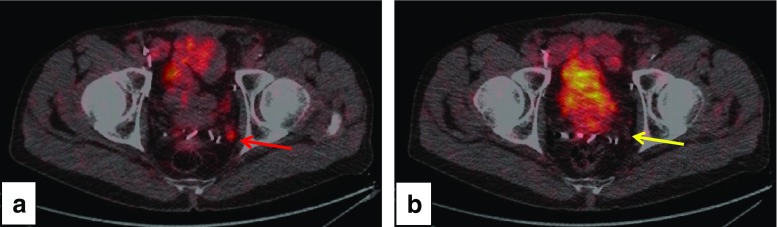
Potential effect of bicalutamide on ^68^Ga-PSMA-11 PET-uptake. On initial PET/CT (a, *red arrow*) moderately increased tracer accumulation with a SUV_max_ of 4.47 in a LN (5.7 mm in diameter) was found in the region of the left internal iliac vessels as shown on axial fused PET/CT-images. On follow-up PET/CT performed 7 days after initiation of bicalutamide (160 mg/day; 1 week), no pathologic tracer accumulation was found in the left iliac LN, which morphologically remained unchanged (b; *yellow arrow*). PSA decreased from 0.94 to 0.18 ng/ml under treatment with bicalutamide
